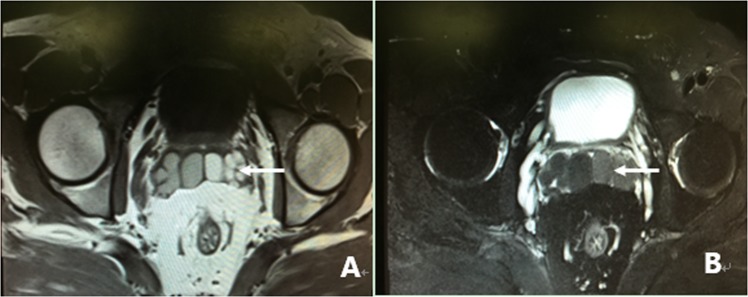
MRI image of EDO. (A) The image shows obvious dilatation or enlargement in both sides of the SV. The SV presents intermediate-high signal intensity on T1WI. (B) Both sides of the SV present as low signal intensity on T2WI. This pattern suggests that EDO is accompanied with a fresh hemorrhage in both sides of the SV.
